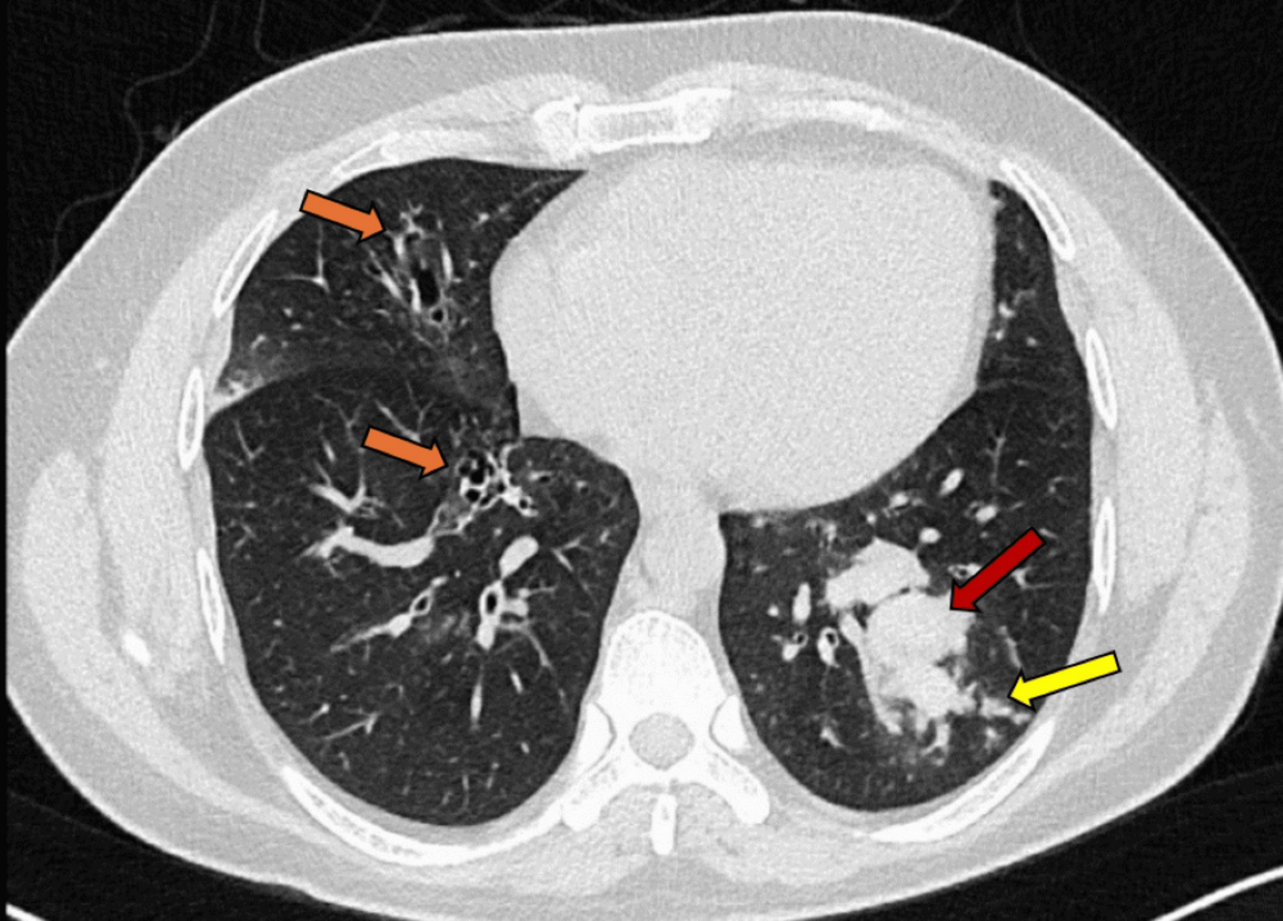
The axial image of the chest CT in the lung window shows bilateral central and peripheral bronchiectasis (orange arrows), as well as a mass-like lesion in the left lower lobe (red arrow) with adjacent small nodular opacities (yellow arrow).
